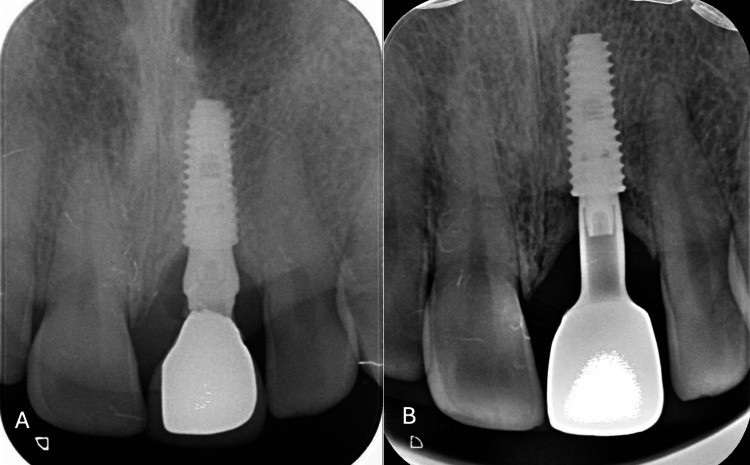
(A) Periapical X-ray at the beginning. (B) Periapical X-ray at the 30-month follow-up
